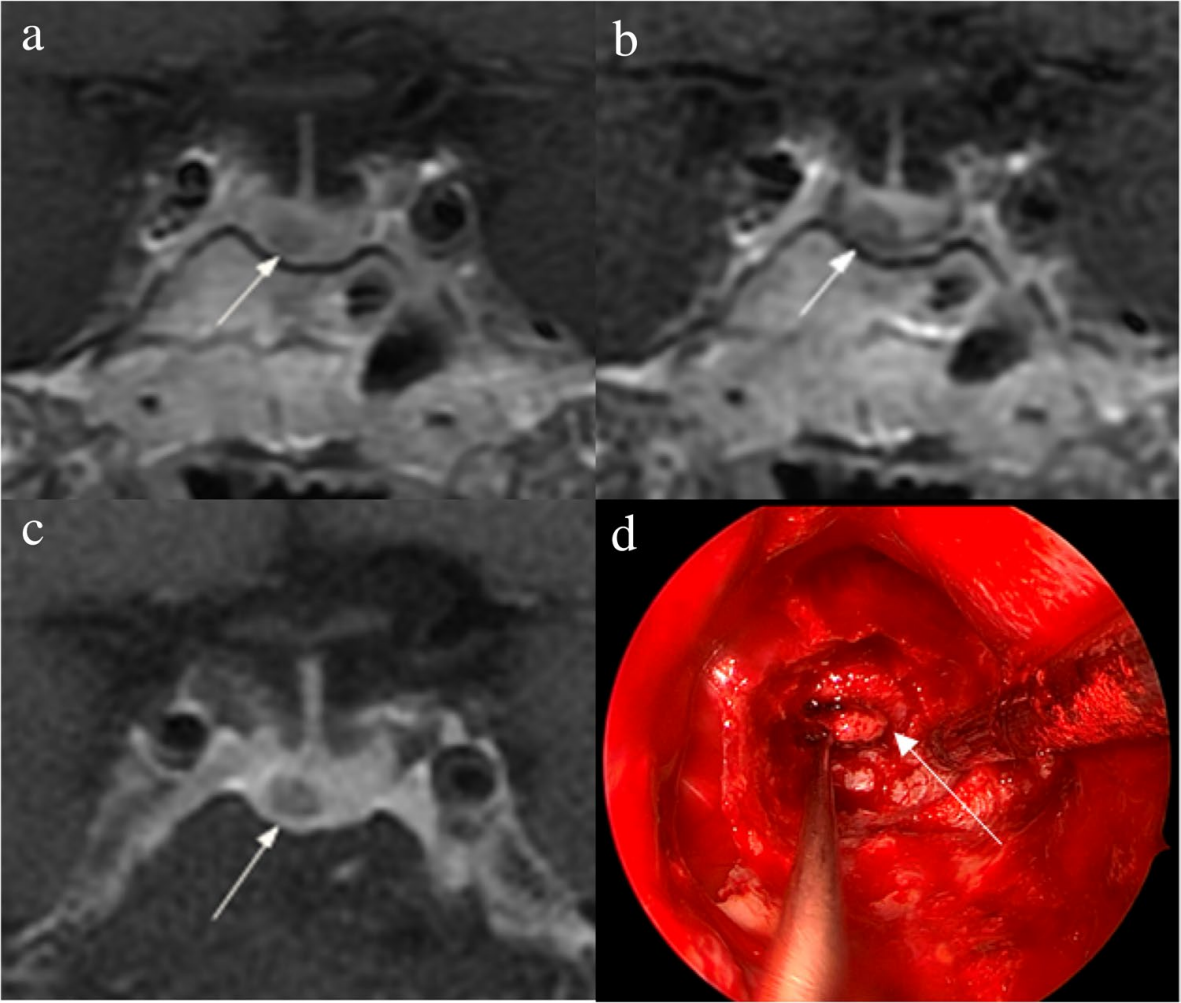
Images in a 56-year-old man with Cushing’s disease. The 5-mm pituitary microadenoma (arrow) can be identified on (a) coronal contrast-enhanced T1-weighted image and (b) coronal dynamic contrast-enhanced T1-weighted image obtained with two-dimensional (2D) fast spin echo (FSE) sequence, but the margin is not fully delineated. The lesion (arrow) is well delineated on (c) coronal contrast-enhanced T1-weighted image on high-resolution MRI obtained with 3D FSE sequence. d Intraoperative endoscopic photograph during transsphenoidal surgery after exposure of the sellar floor shows a round pituitary microadenoma (arrow)
